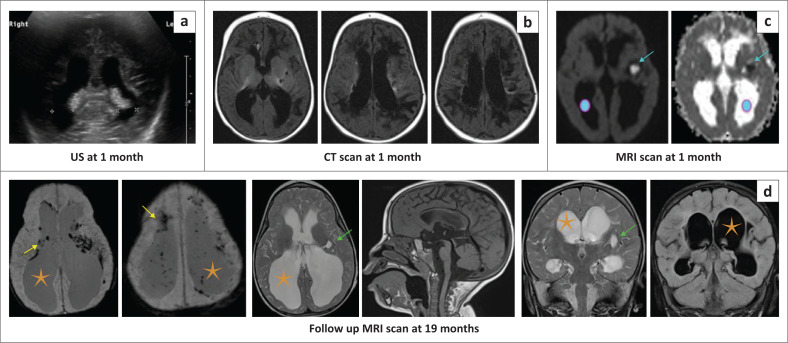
(a-d) In utero toxoplasmosis, rubella, cytomegalovirus, herpes (TORCH) (*cytomegalovirus*) infection with neonatal hydrocephalus and periventricular hyperechogenicity on sonar. Follow-up CT at 1 month shows periventricular calcification and increasing ventricular prominence. Putaminal restricted diffusion (cyan arrows) and slightly larger ventricles (O) were shown on the MRI studies performed at 1 month of age. Follow-up MRI at 19 months reveals more marked hydrocephalus (orange star), periventricular calcification (yellow arrows) and basal ganglia spongiosis (green arrow) related to prior secondary ischaemic injury. These sequential complications of *TORCH* infection are important in distinguishing it from hypoxic-ischaemic brain injury.
